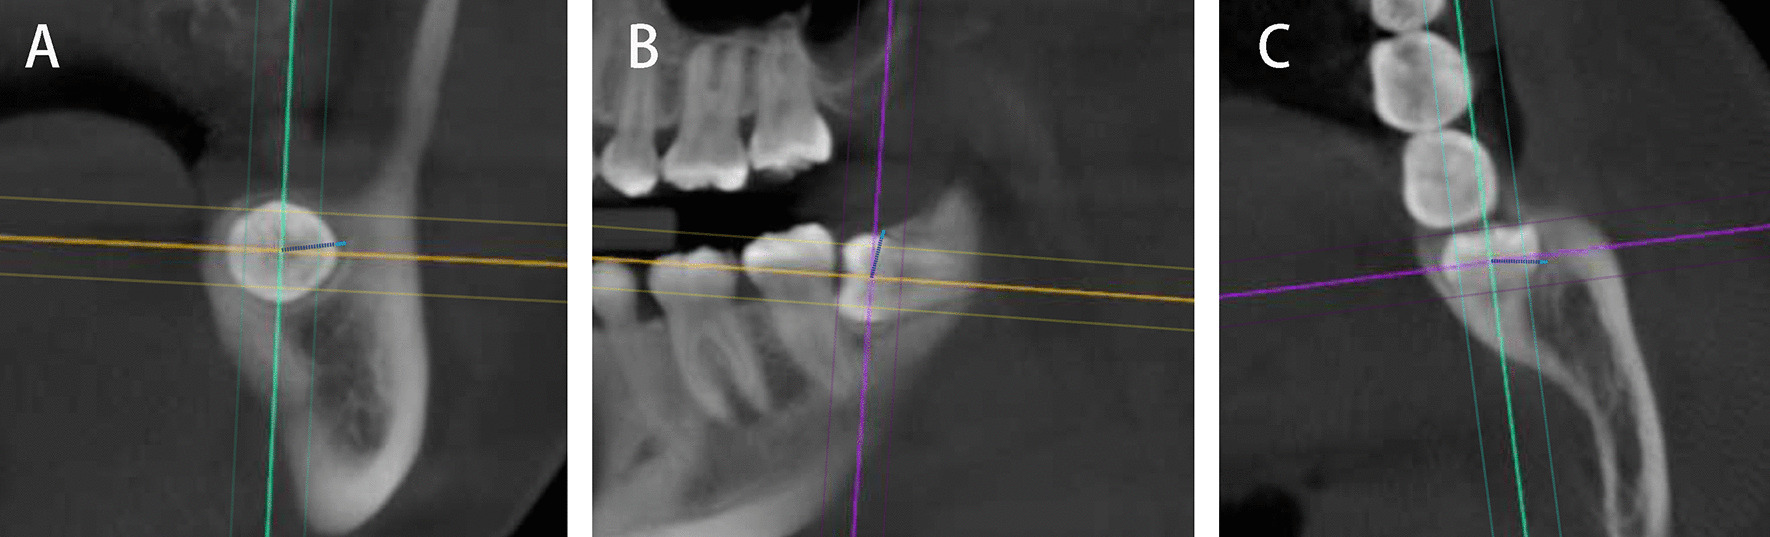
Measurement of the maximum width of the dental follicle around the crown by CBCT. A The measurement in coronal section. B The measurement in Sagittal section. C The measurement in horizontal section. The blue line indicates the width of the dental follicle
